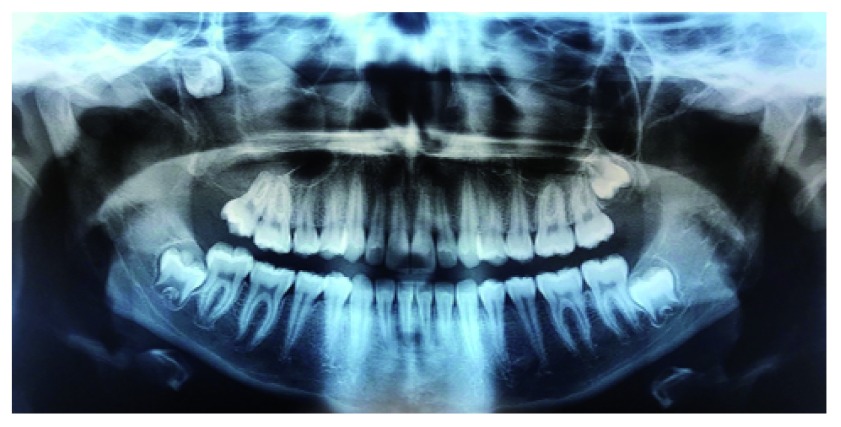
Preoperative panoramic radiograph showing an ectopic eruption of the right maxillary third molar in a superior position near pterygomaxillary fissure inside the right maxillary sinus and surrounded by well-defined hyperdense lesion obliterating the right maxillary sinus cavity.
